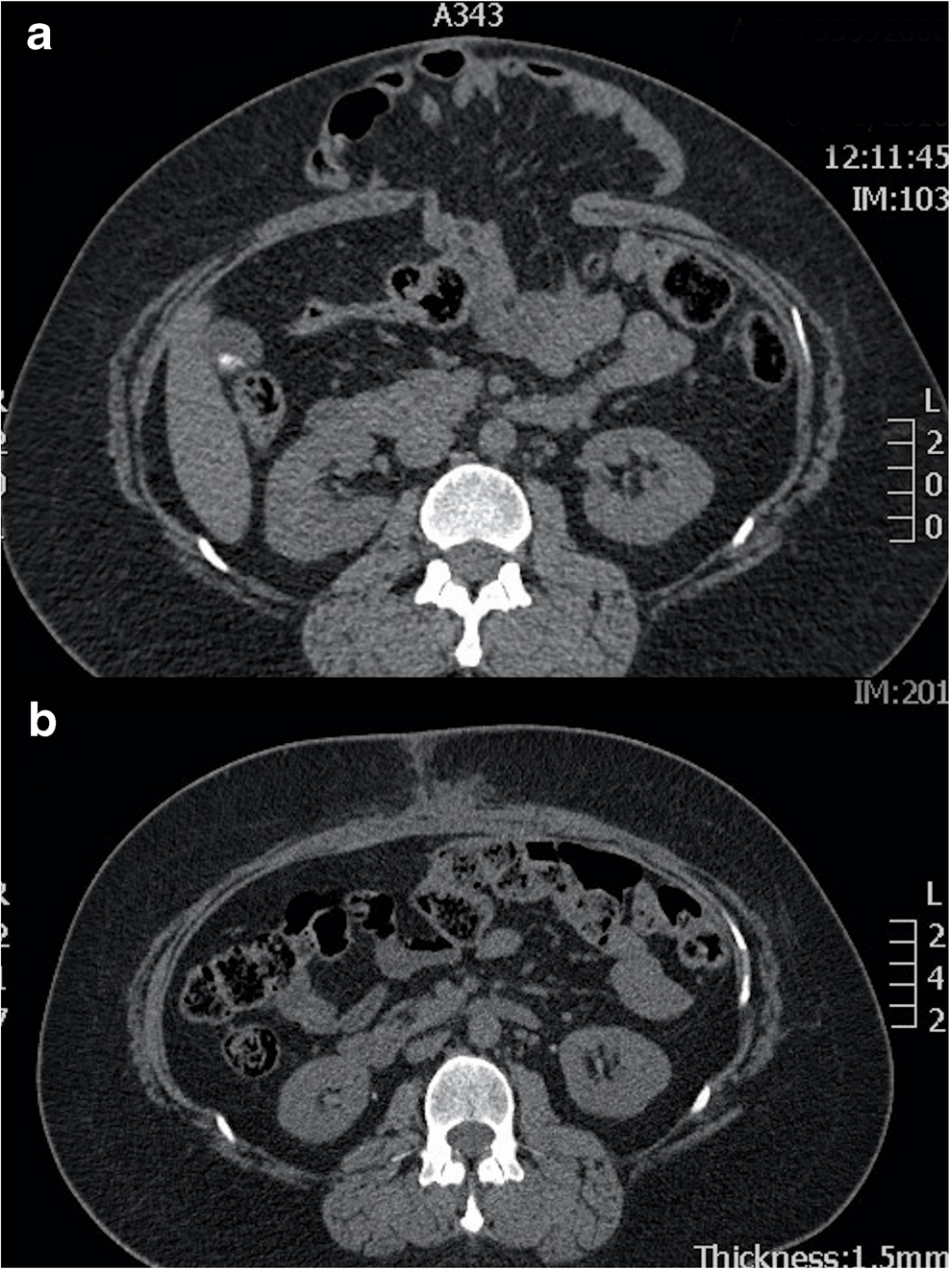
a Preoperative axial CT showing a ventral hernia containing small bowel. b Postoperative axial CT showing ventral hernia repair achieved by apposition of the rectus muscles in the midline combined with an intraperitoneal mesh
